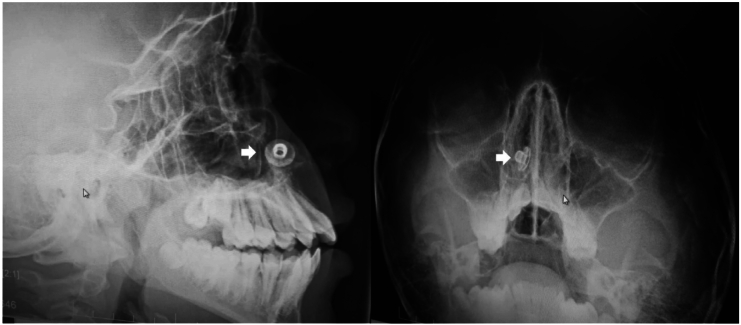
Lateral and frontal X-rays views showing a circular, radiopaque object situated along the nasal floor *(arrows)*.
